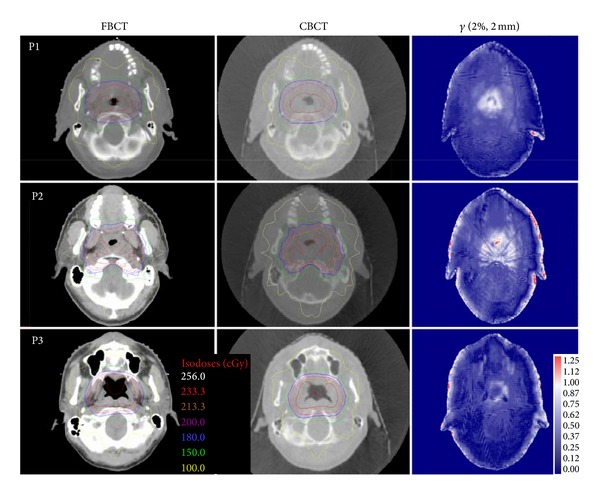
Comparison of dose distribution and gamma values on the transversal plane at the isocenter position between the two plans based on FBCT and CBCT images for three patients (P1–P3). On the right (the third column) are the gamma comparison results (2%, 2 mm) of these two plans. The red areas denote pixels with gamma values greater than 1.
